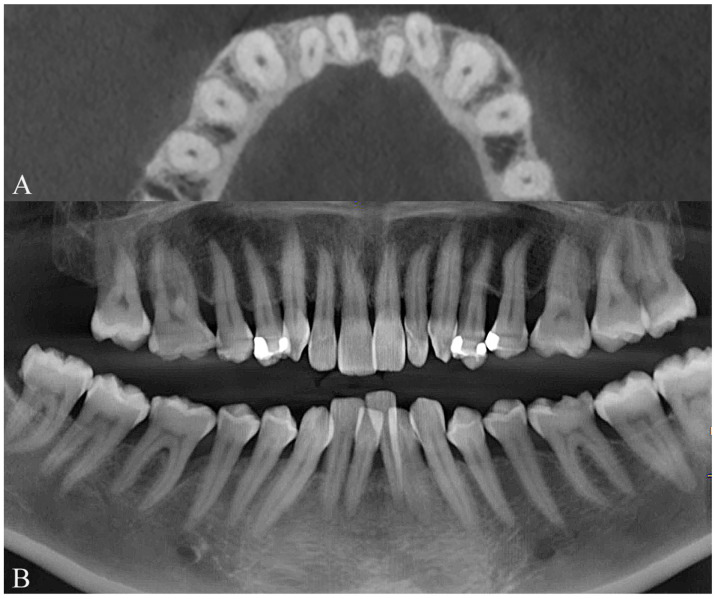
Initial radiographic records of Clinical Case 1. (A) CBCT sections of the mandibular anterior region. (B) Panoramic radiograph reconstructed from the CBCT.
